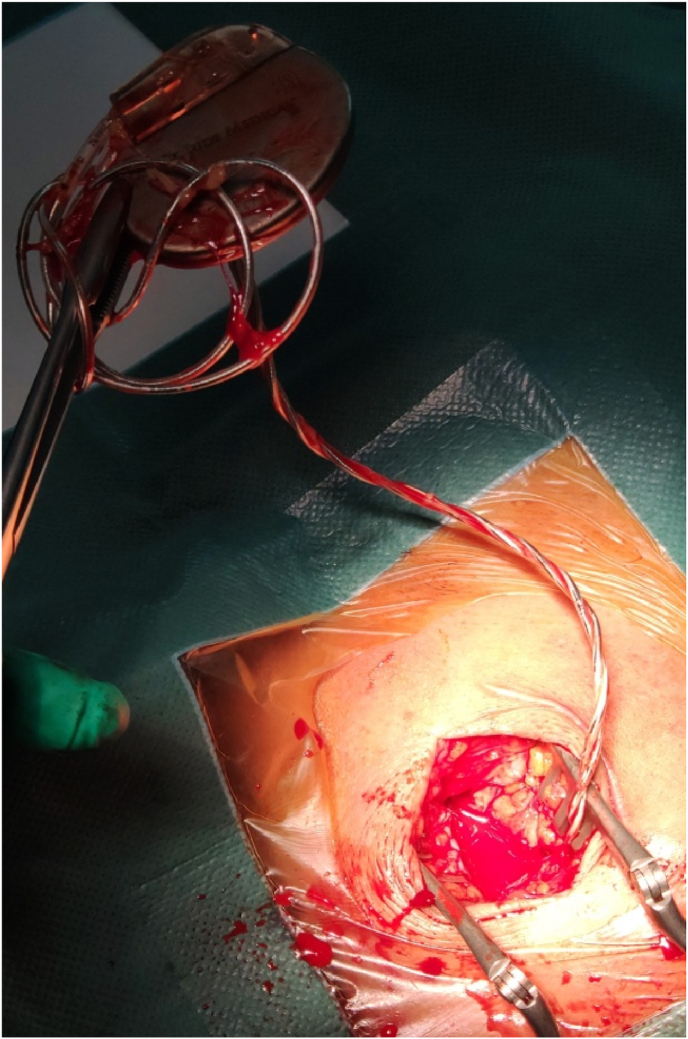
Peroperative picture confirm the findings of the chest X-ray, Both leads were found tangling and wrapped around the pacemaker generator without any signs of structural damage.
